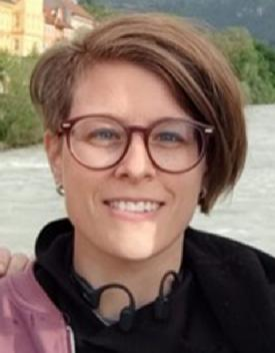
Dr Anna Jöbstl earned her medical degree from the Medical University of Vienna in 2017. From 2018 to 2024, she was a radiology resident at KH Spittal an der Drau and LKH Innsbruck. She has been working as a certified radiologist at LKH Innsbruck from January to December 2024 and at MRI-Institute Spittal an der Drau since January 2025.
